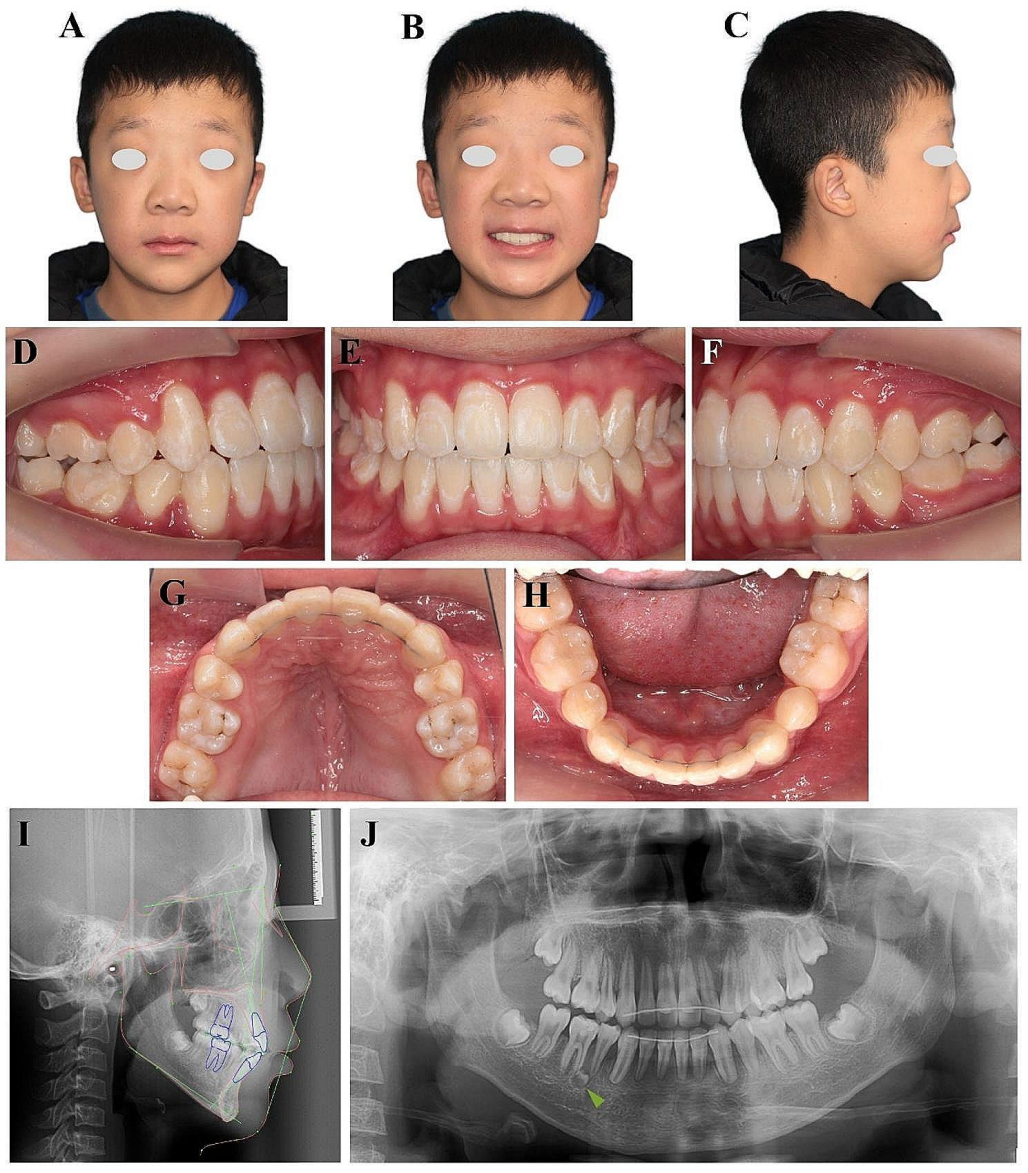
Posttreatment examinations of this patient. (A-C) Posttreatment facial photographs. (D-H) Posttreatment intraoral photographs. (I) Posttreatment lateral cephalogram. (J) Posttreatment panoramic radiograph. Notably, a new supernumerary tooth (indicated by the green arrowhead) emerged post to the teeth extraction surgery
